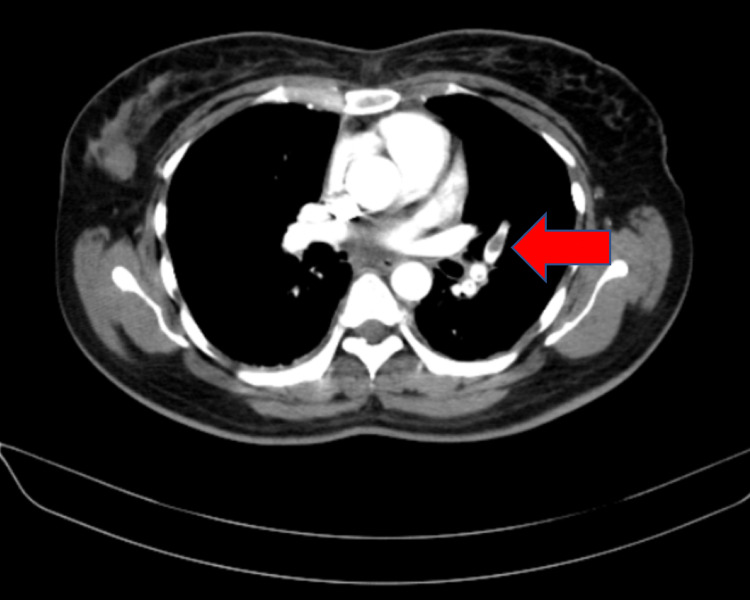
CT pulmonary angiography showing pulmonary embolism CT-Computed tomography
